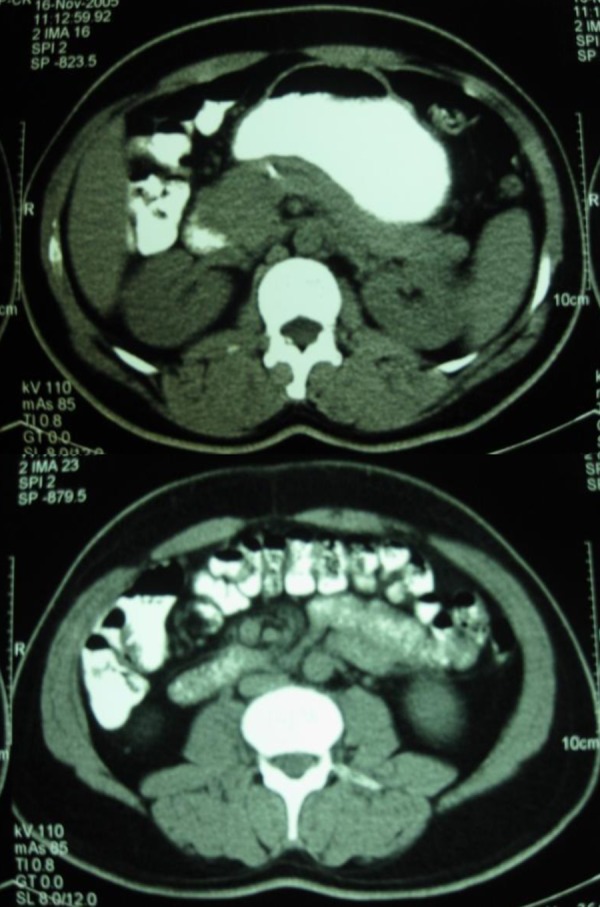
Abdominal CT scan showing pancreatitis (black star) and a metallic lesion in the course of the main pancreatic duct (white arrow)
